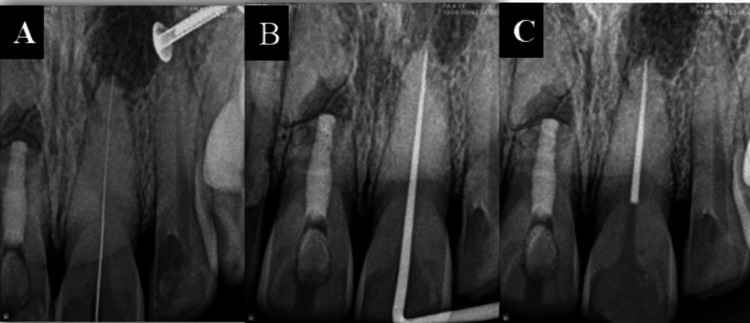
(A) Working length radiograph, (B) master cone verification radiograph and (C) obturation radiograph
